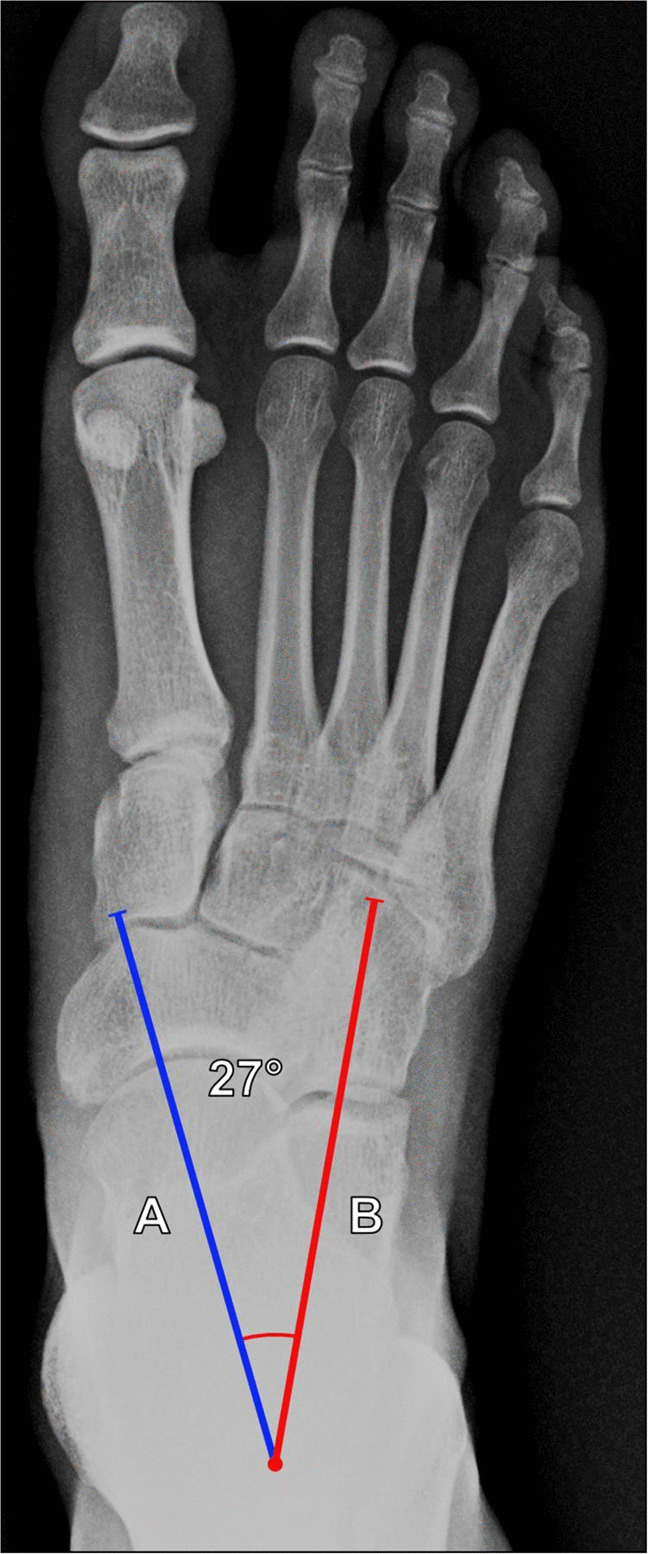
Measurement of the AP talocalcaneal (Kite) angle on DP weightbearing radiograph of the foot. The angle is formed between a line (A, blue line) along the talar axis and a line (B, red line) along the calcaneal axis. Normal values range between 25° and 40°. Angles < 25° suggest hindfoot varus, and those > 40° suggest hindfoot valgus
